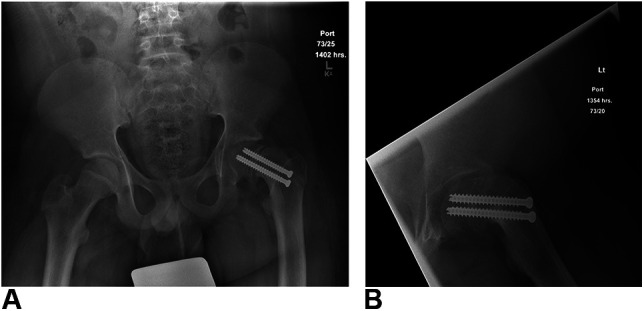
A, Anterior-posterior pelvis and (B) left hip lateral postoperative radiographs showing two screws traversing the physis of the severe SCFE with screw placement on the anterolateral femur to accommodate the 3D morphology of the SCFE. SCFE = slipped capital femoral epiphysis.
